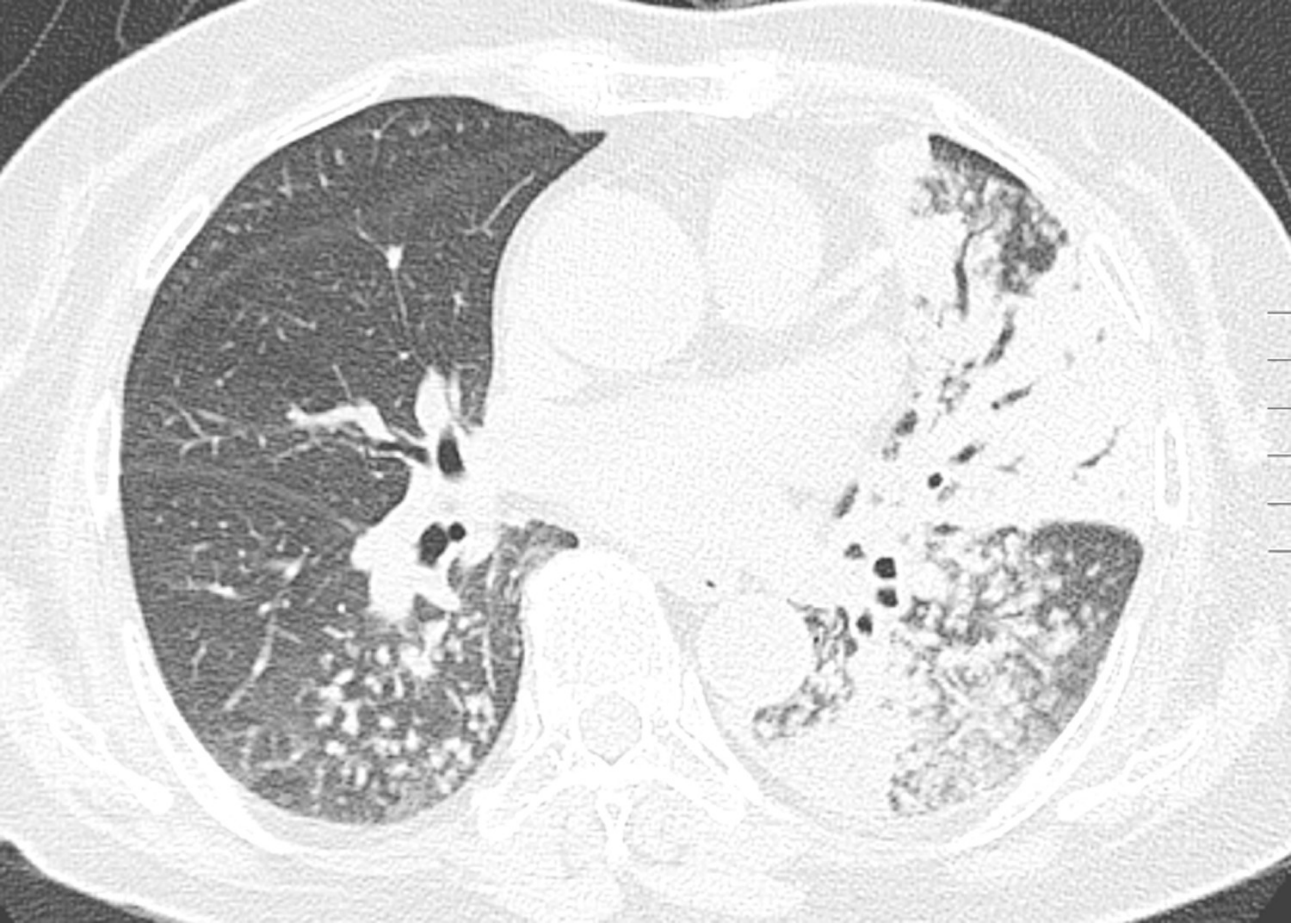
Primary multidrug-resistant tuberculosis with 58-year-old woman. Lung window setting of thin section CT shows centrilobular nodules and tree-in-bud lesions in right lower lobe, lingula and left lower lobe. Lobar or segmental consolidation and ground glass opacity are seen in lingula.
